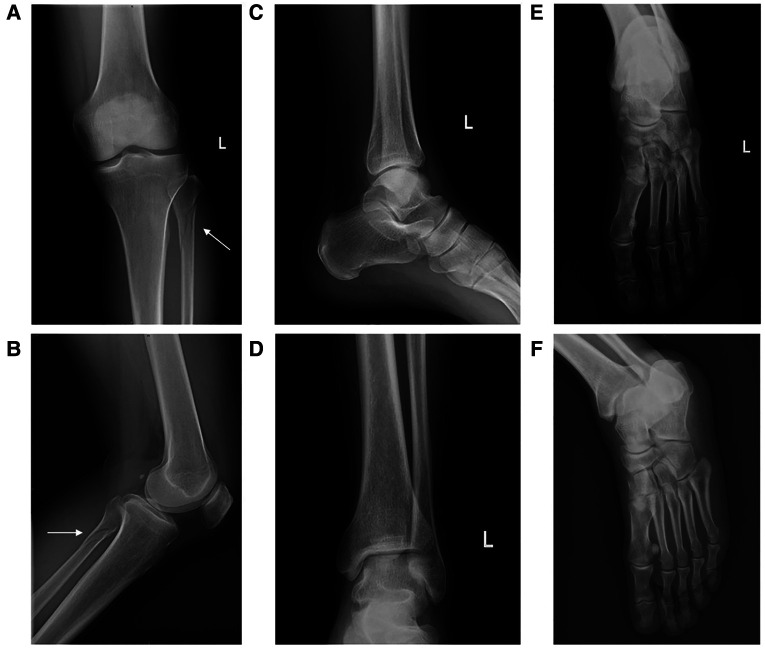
The initial x-ray images of the proximal fibula (A,B), the ankle (C,D), and the foot (E,F).
